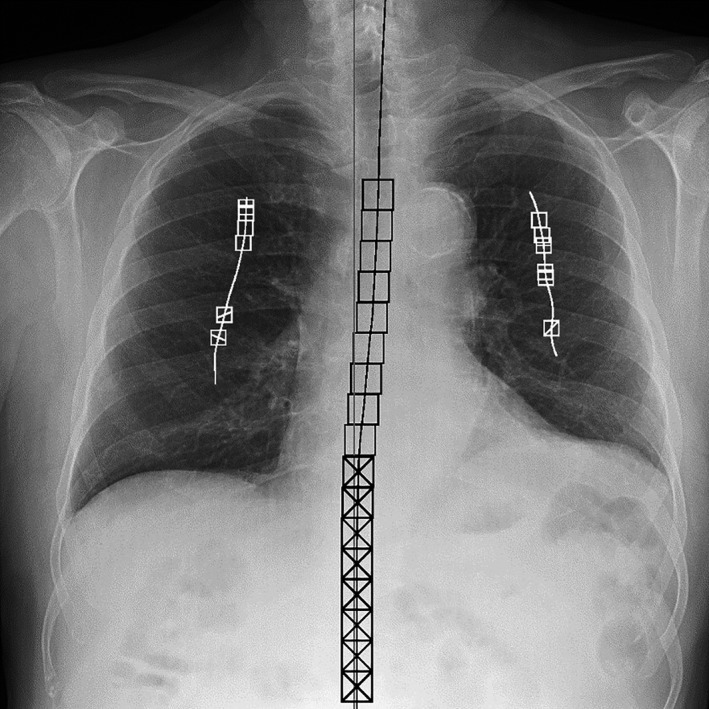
ROI placement example on a PA chest radiograph. White squares without lines are Lung ROIs, while those with a line indicating rib edge crossings are Rib/Lung ROIs. Black squares without crosses are mediastinum ROIs, and those with crossed lines are subdiaphragm ROIs.^24^
